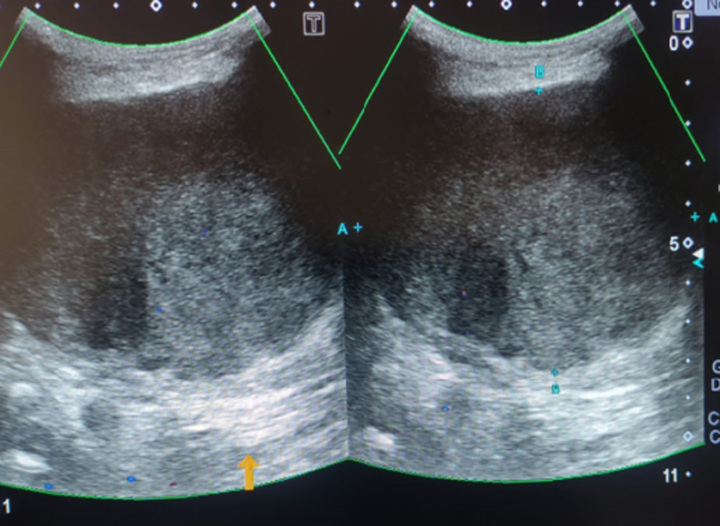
Ultrasound showing a well-defined hypoechoic lesion in the subcutaneous tissue of the left side of the back, approximately measuring 9.3×7.0 cm.
